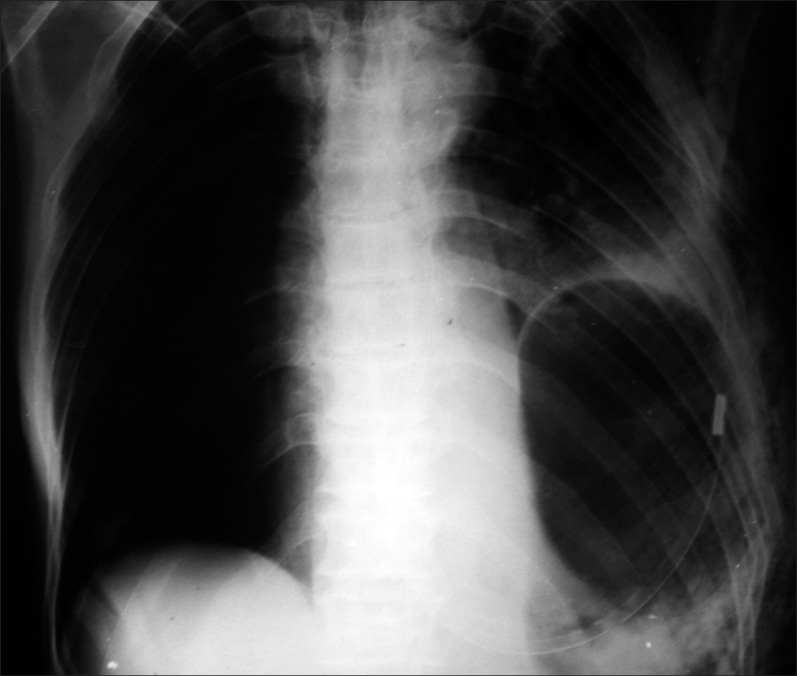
Pre-operative X-ray chest showing left diaphragmatic hernia
